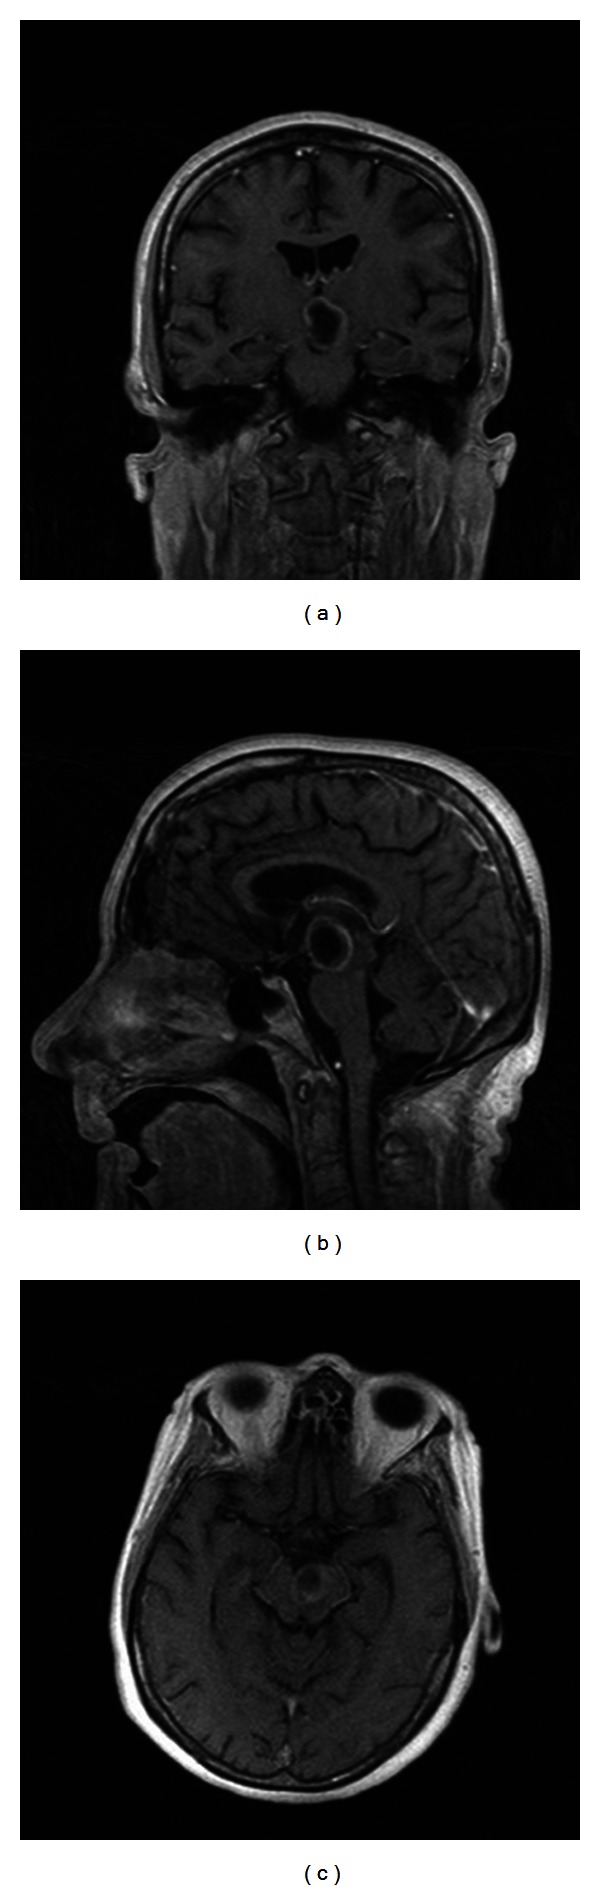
Cystic metastasis. Rim-enhancing hypointense mesencephalic lesion revealed by postcontrast T1-weighted MRI images ((a): coronal, (b): sagittal, and (c): axial slices). Department of Neurology, Colentina Clinical Hospital brain imaging archive.
